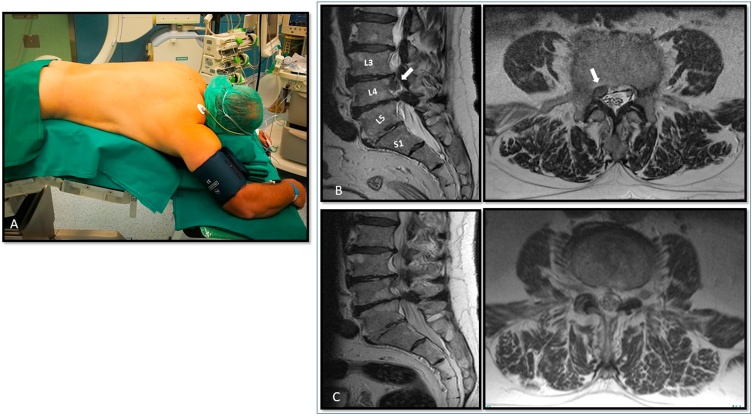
A. patient positioned on a prone position on the operating table. The patient is awake, only mildly sedated, waiting for the local anesthesia and the procedure to start. B. Preoperative sagittal (*left*) and axial (*right*) T2-weighted lumbar MRI scan showing a right L3-L4 paramedian disc herniation caudally migrated. C. Three-months postoperative T2-weighted lumbar MRI scan shows the satisfactory outcome of the procedure and the lack of signal intensity suggestive for pedicle damage or fracture.
